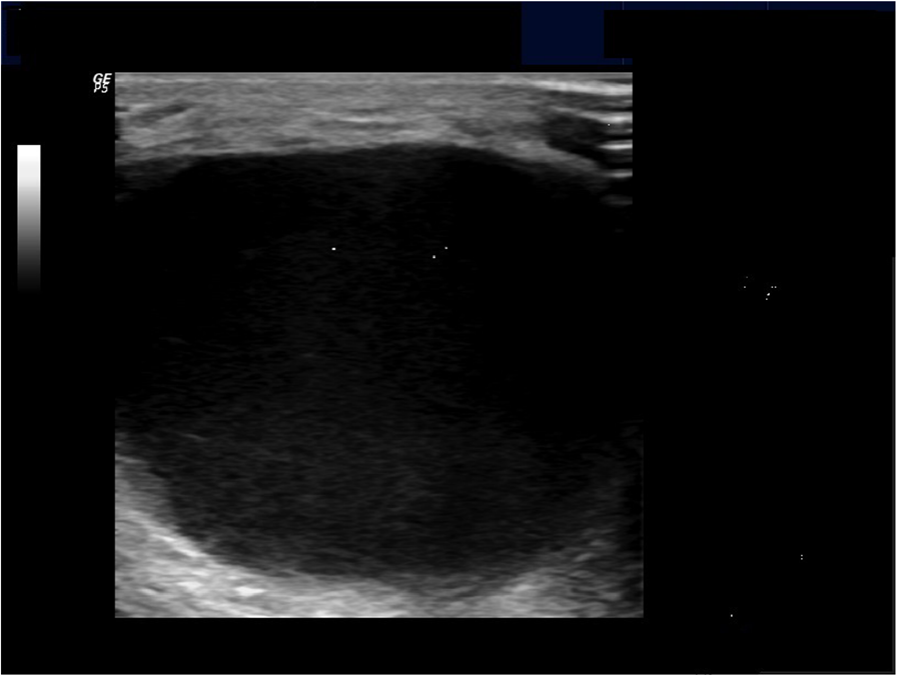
There was a huge fluid collection between fascial compartments and muscle layers on ultrasonography
